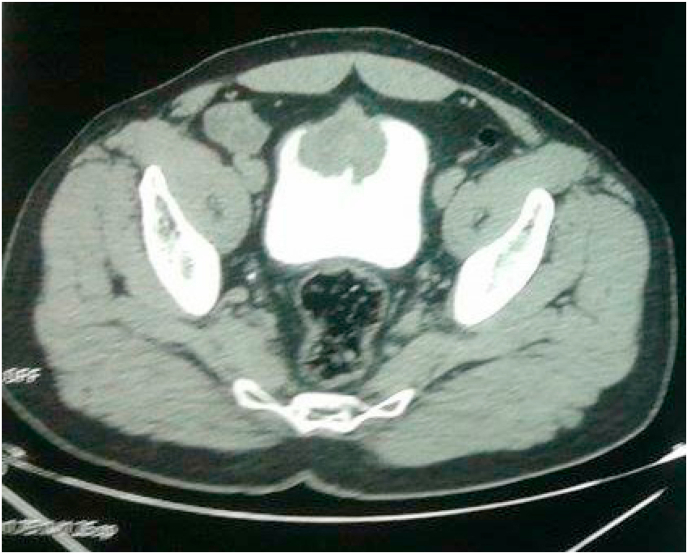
Abdomino-pelvic CT scan showed budding wall thickening of the anterior wall of the bladder.
